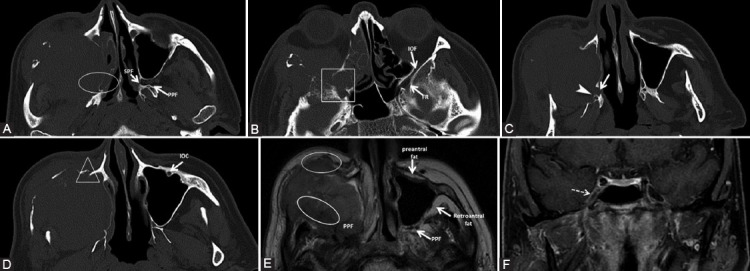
The patient presented with inflamed infraorbital and cheek region for about 3 weeks, no prior history of malignancy. Diagnosed squamous cell carcinoma of the maxillary sinus with orbital and ethmoidal extension. Bone window axial computed tomography scans (A, B, C, D) show direct tumor invasion of pterygopalatine fossa and sphenopalatine fissure (ellipse), as well as invasion of the inferior orbital fissure and foramen rotundum (rectangle). The tumor also invades the right hard palate with the enlargement of the greater (arrow) and lesser (arrowhead) palatine canals, and the infraorbital canal (triangle), compared to the normal left one. Axial T2-weighted image (E) shows the right PPF, pre- and retroantral fat obliteration (ellipses) versus the normal left side. T1-weighted fat-saturated coronal image (F) with contrast medium demonstrates the contrast enhancement of the right Meckel’s cave (dashed arrow), not seen on CT, suggesting the involvement of the trigeminal ganglion.
